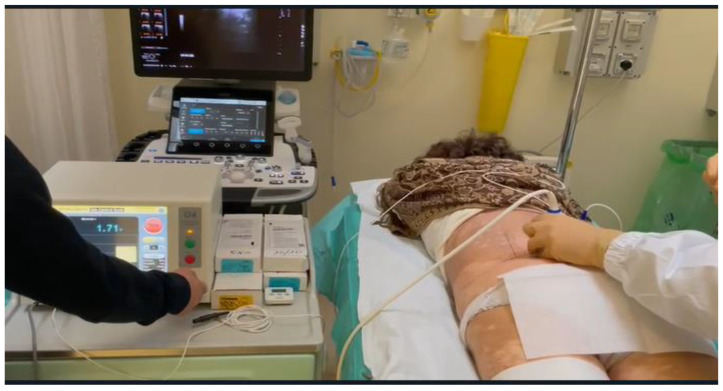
Ultrasound-guided pulsed radiofrequency treatment of L5 dorsal roots ganglion in a 75-year-old female patient with persistent low back pain and chronic sciatica.
